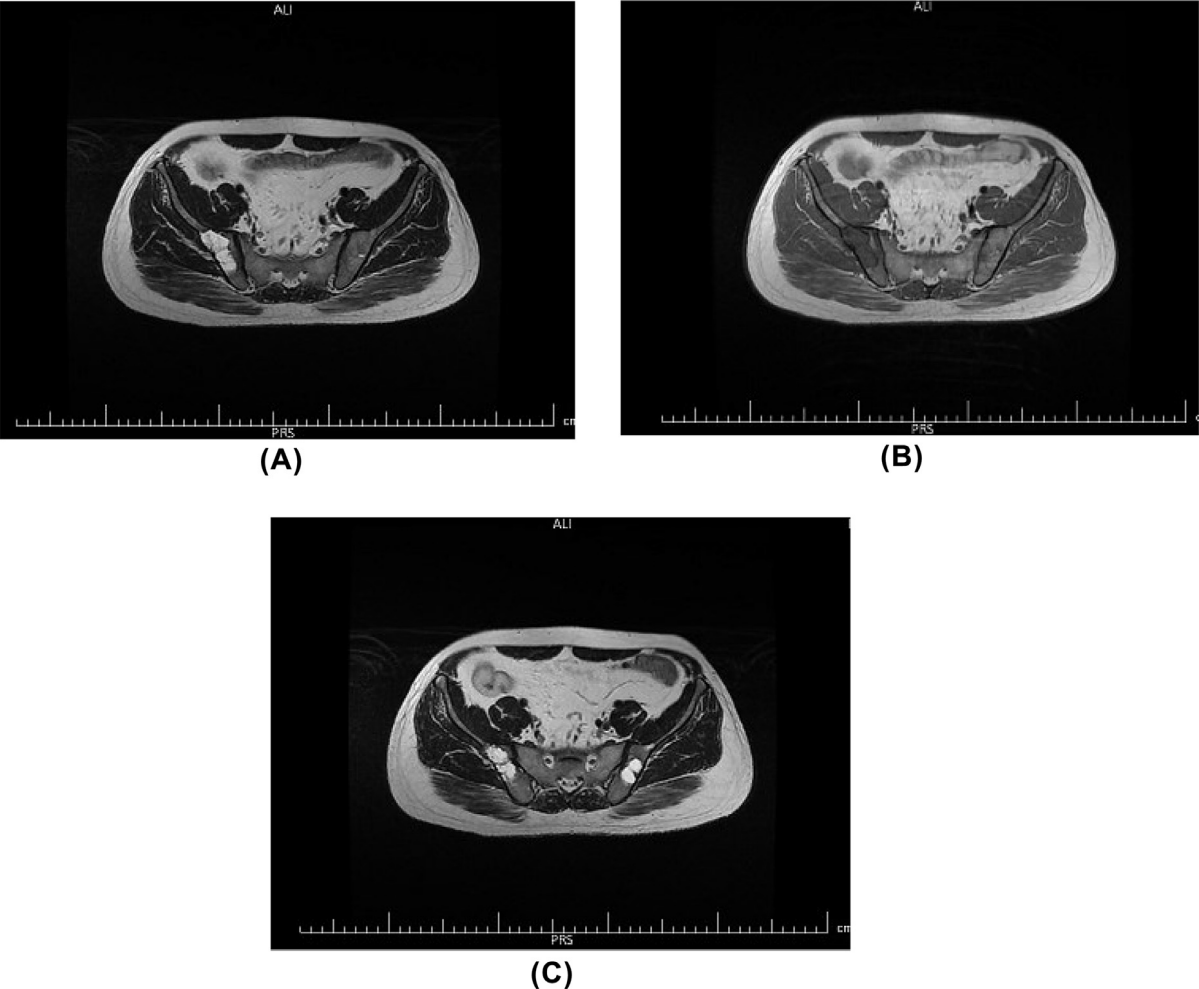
(A–C) In the pelvic MRI, there were 2 well-defined multiseptated cystic lesions in bilateral iliac bones which show high signal intensity on T2 and low signal intensity on T1 sequence. No cortical disruption or soft tissue component was visible. Regarding patient history, findings are compatible with macro cystic lymphangioma.
